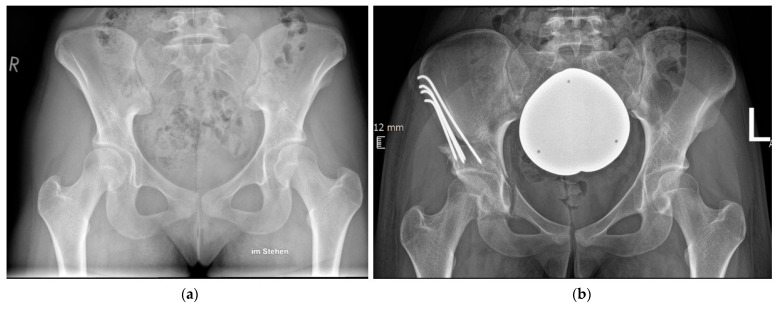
(a) Preoperative a.p. radiograph before PAO. (b) Postoperative radiograph after PAO and fixation with K-wires.
